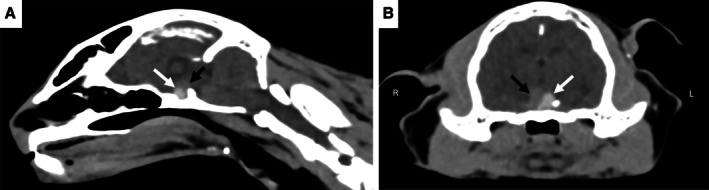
Sagittal (A) and transverse (B) CT of the head. White arrows point the suprasellar lesion, black arrows the apoplexy region.
